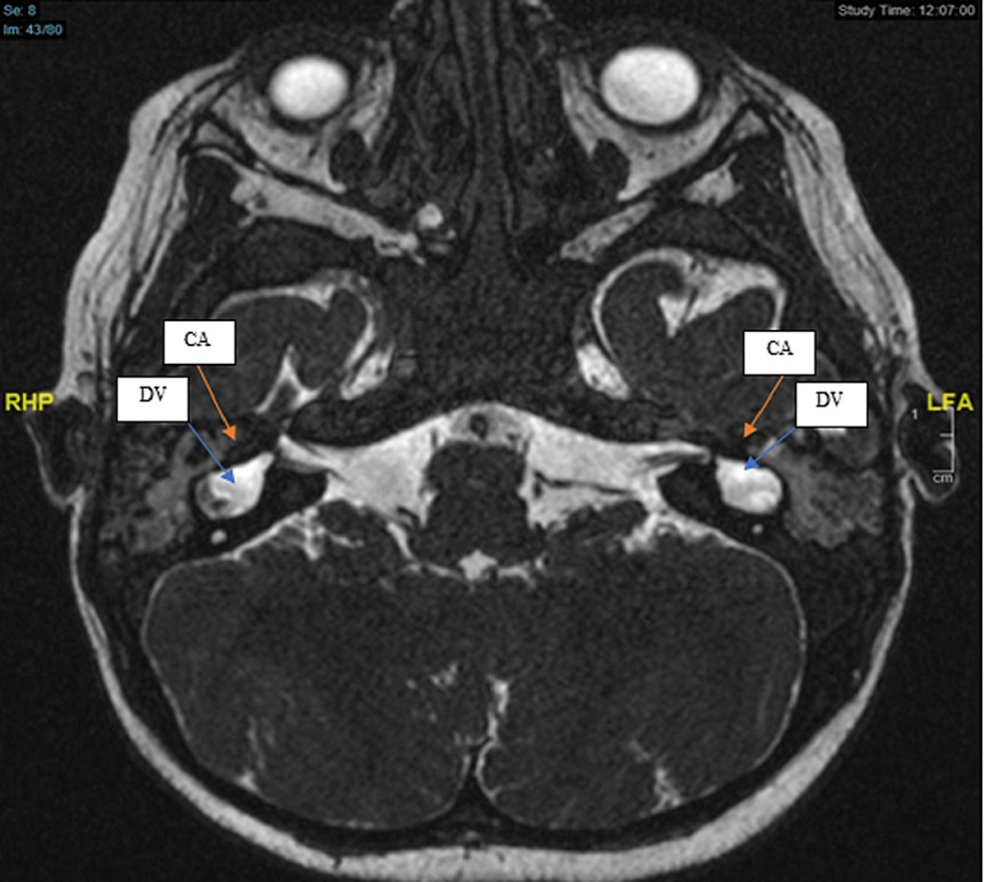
MRI internal auditory canal showed bilateral cochlear aplasia (CA), the dilated vestibule (DV).
